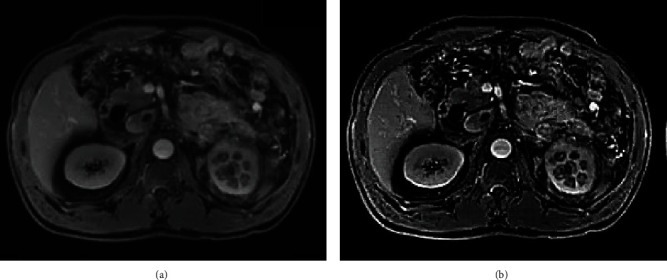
Imaging data of a 45-year-old male patient with left kidney disease. A was the original BOLD-MRI scan, and B was the image segmented by the KFCM algorithm.
